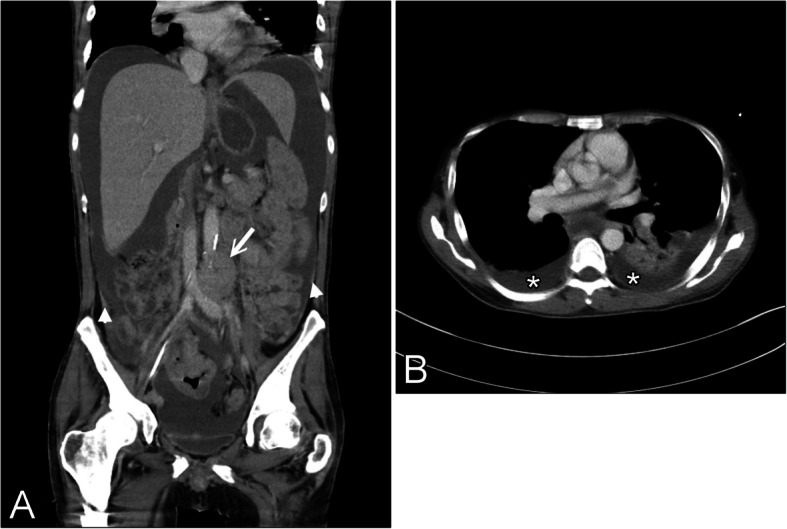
A 41-year-old, HIV-infected male patient (Patient no. 9) underwent imaging studies after diagnosis. Post-contrast-enhanced coronal CT images of the abdomen (A) and axial CT images of the lower chest (B) demonstrate a large volume of ascites and bilateral pleural effusions (*). An enlarged para-aortic lymph node (arrow) and peritoneal nodular thickening (arrowheads) are observed. Partial collapse of the left lower lobe of the lung is also noted
